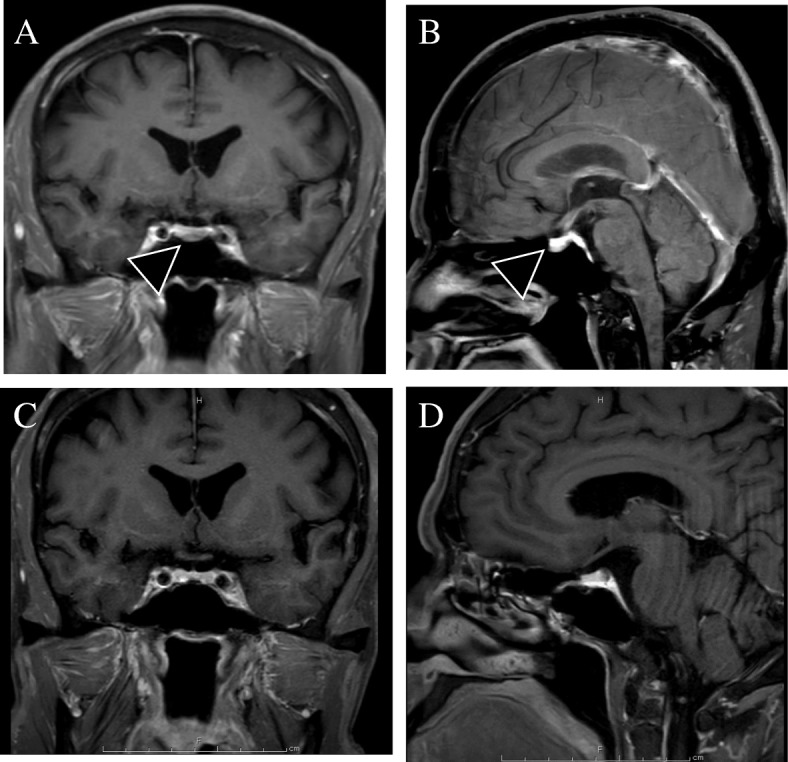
Gadolinium-enhanced pituitary magnetic resonance imaging. a Coronal section. b Sagittal section (before surgery). c Coronal section. d Sagittal section (after surgery). Arrow showed a pituitary adenoma 11 mm in diameter
